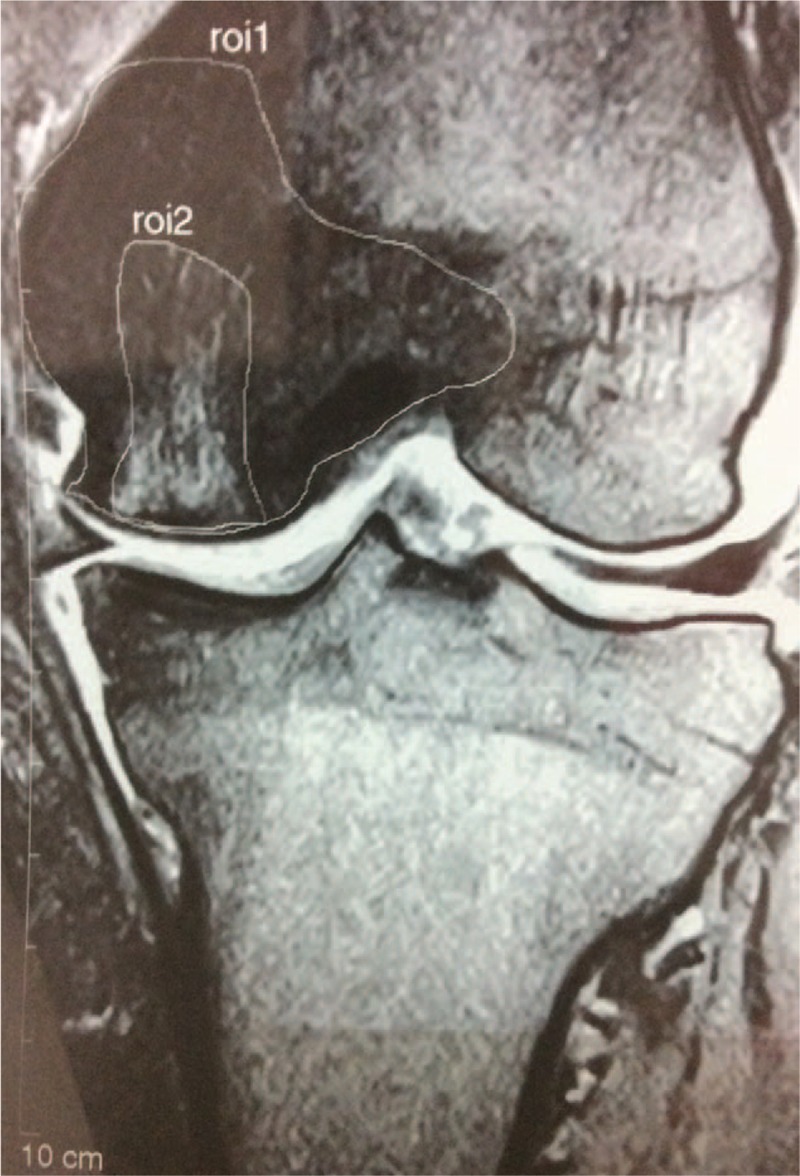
Case 1 in the initial phase. MRI shows low and high-intensity areas that identify the SONK lesion. MRI = magnetic resonance imaging, SONK = spontaneous osteonecrosis of the knee.
